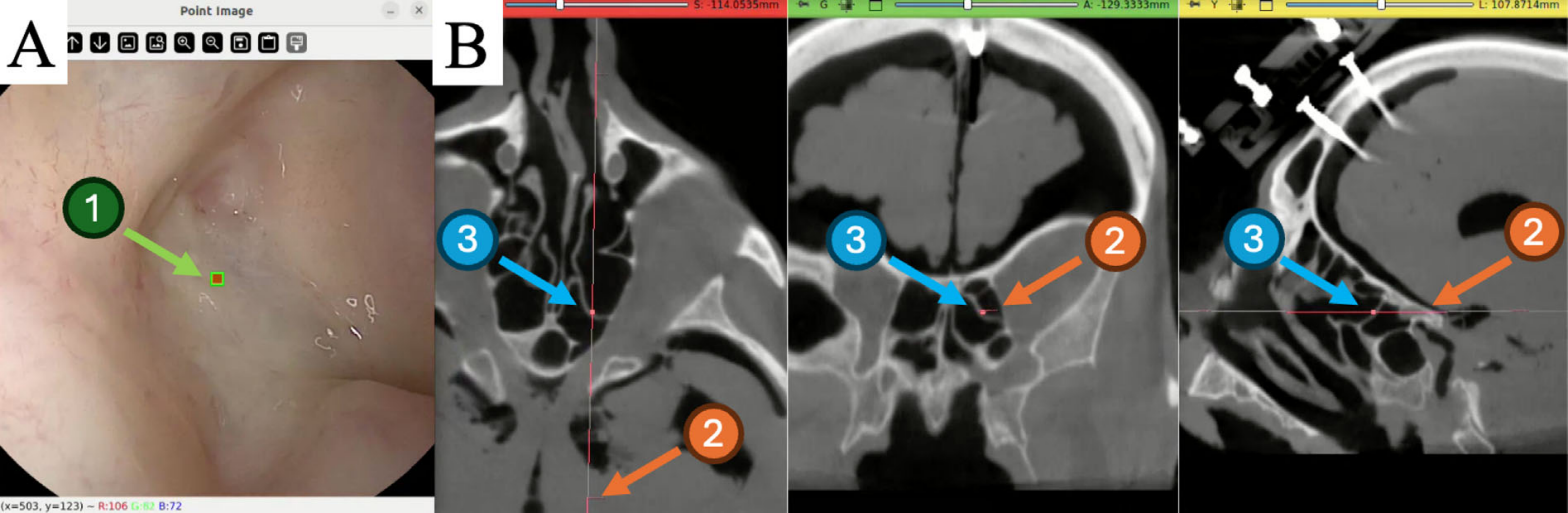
(A) Image endoscope reference view and (B) CT slices of the explored anatomy. In this proof of concept, (1) shows a visual reference in the image that correlates to the CT visual marks. Using the estimated camera pose, we project a virtual line in CT space through the reference (1). This line is indicated by (2). Finally, (3) represents the intersection of the projected line (2) with the tissue. The TRI‐plane view is center at this intersection point and updates its position as the endoscope moves.
